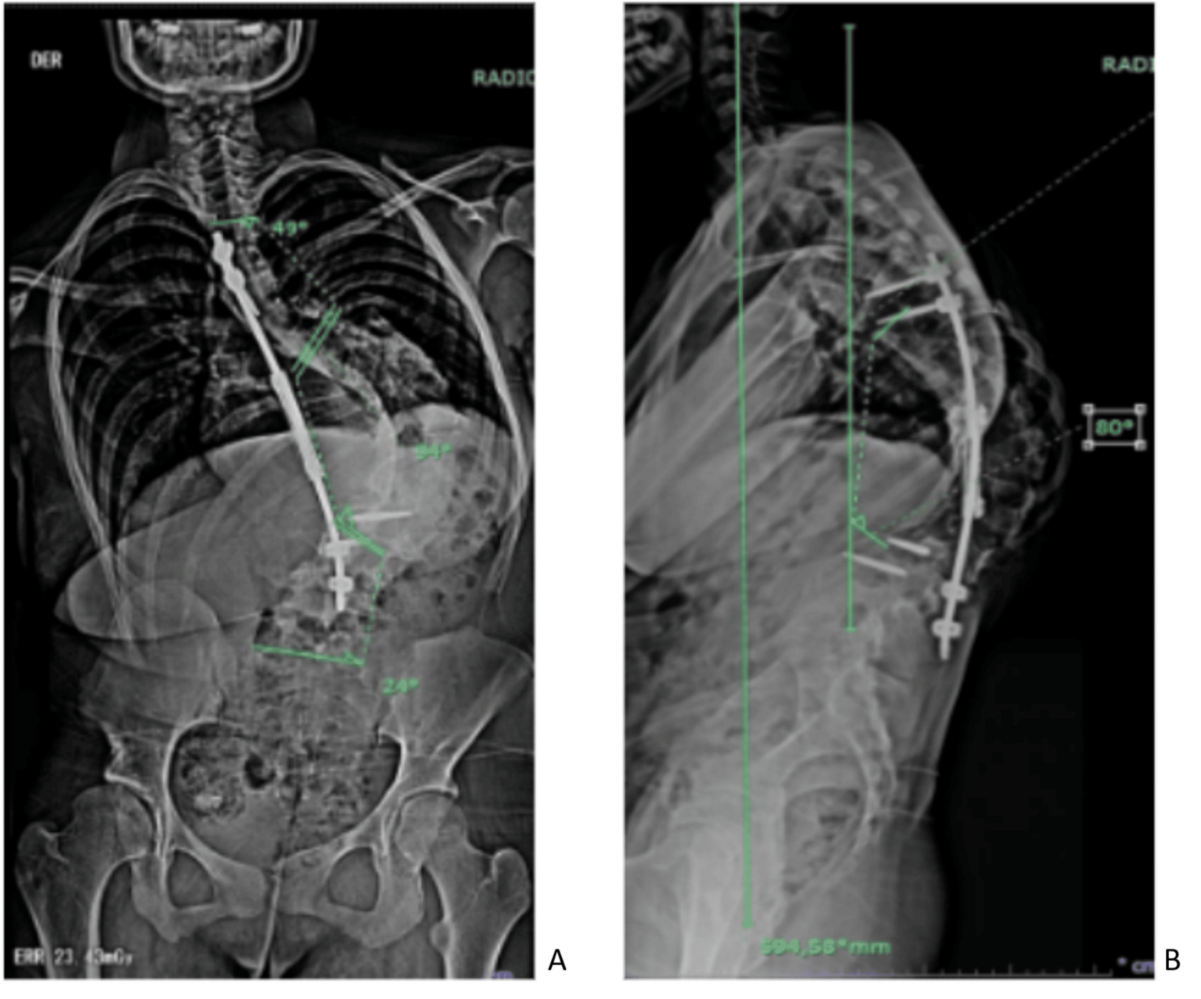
Preoperative anteroposterior (AP) and lateral panoramic spinal radiographs obtained 12 months before surgery. (A) Proximal thoracic curve measuring 49°, main thoracic curve 94°, and thoracolumbar curve 24°, demonstrating right coronal imbalance. (B) Mechanical failure of the lengthening system due to fracture of the distal transpedicular screws. Thoracic kyphosis from T5 to T12 measured 80°, with positive sagittal balance.
